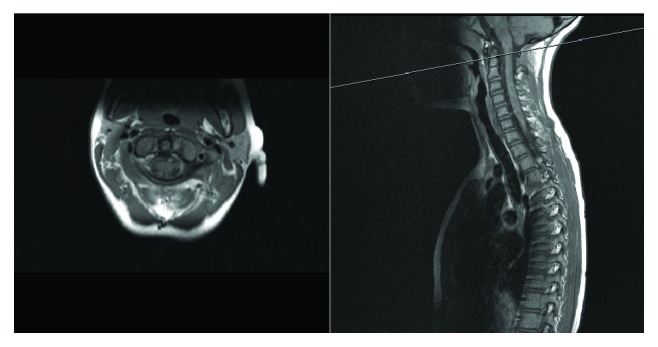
MRI of C-spine T2 stir imaging.
